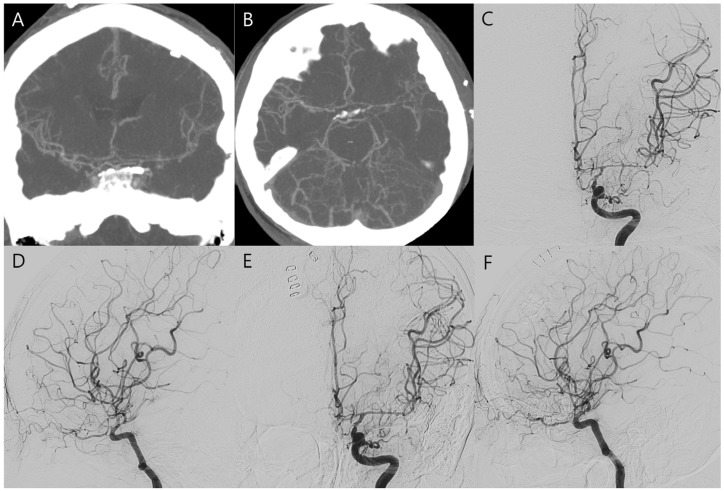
(A–D) On postoperative day 8, CT angiography and subsequent digital subtraction angiography demonstrate severe vasospasm, especially in the left internal carotid artery, left anterior cerebral artery, and left middle cerebral artery. Following intra-arterial nimodipine infusion, the vasospasm improved (E,F).
